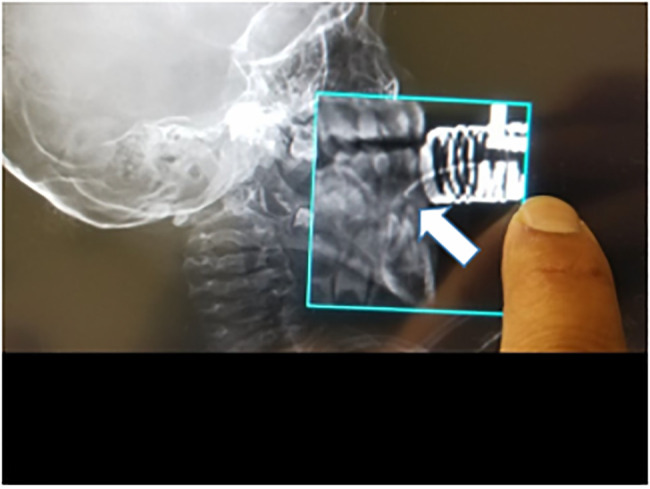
Cranial radiographs identifying foreign body (arrow).
